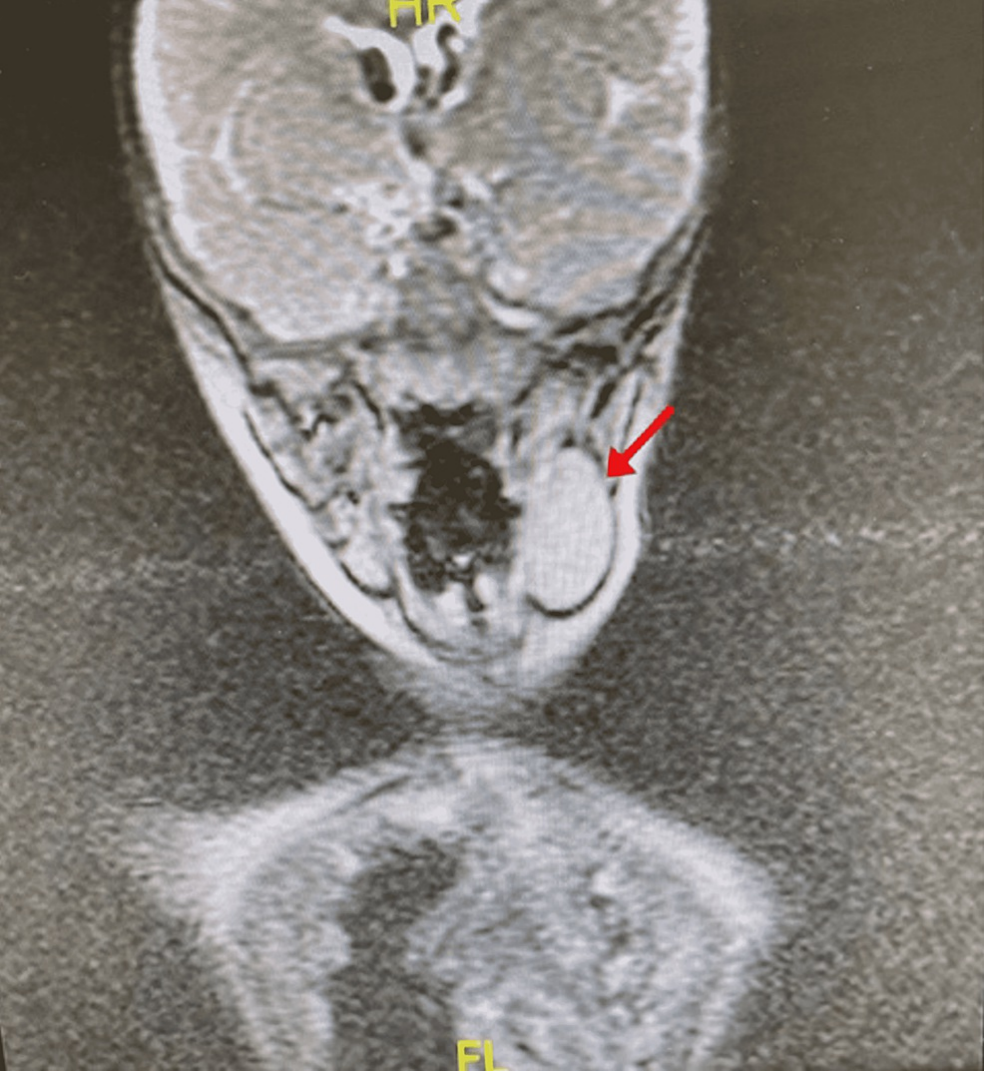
T2 head and neck MRI in coronal view showing isotense mass in the submandibular region suggesting ectopic thymus (red arrow).
